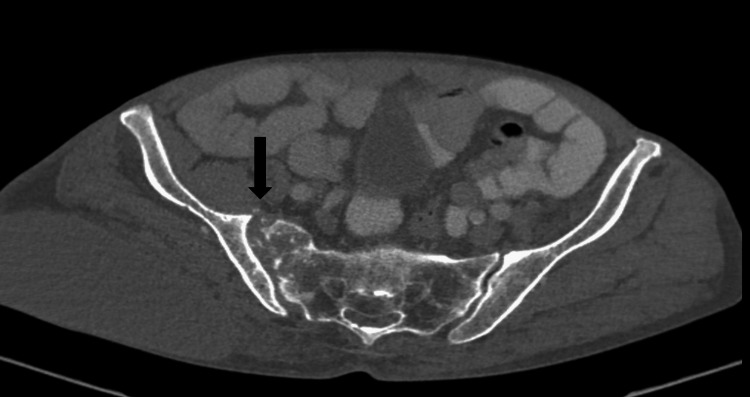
Axial CT of the pelvis, bone window The arrow shows osteolysis of the right sacrum. The left sacroiliac joint shows arthrosis.
